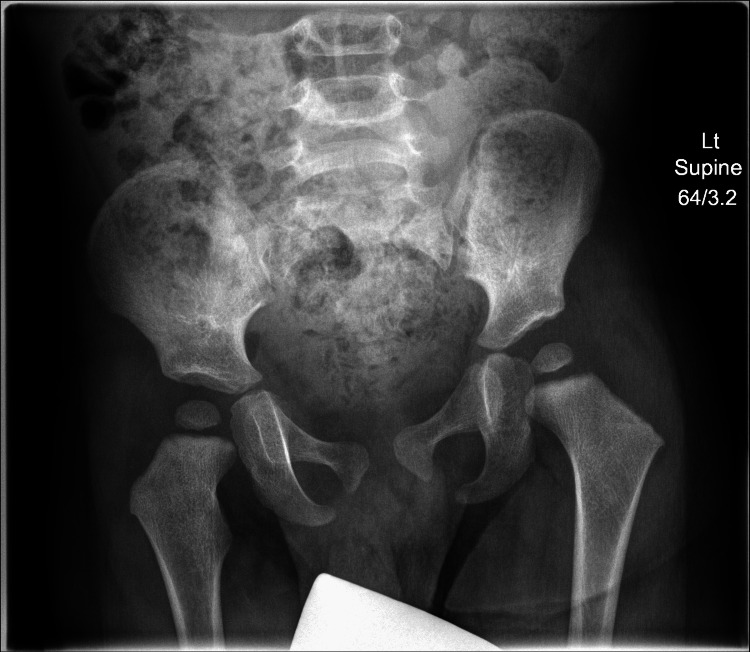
Post-intervention pelvic X-ray. Acetabular index was 25° on the right and 23.1° on the left. Lt: left
